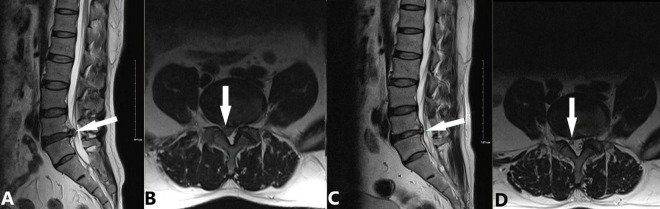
Application of targeted nucleus pulposus chemical ablation for LDH caused by extrusion at the right posterior margin of L4/5. A 45-year-old male patient presented with a 3-month history of lumbar distension and pain, which had progressively worsened in the past week. Considering the extruded nucleus pulposus and its moderate volume, we employed the CT-guided local targeted treatment method proposed by Wu et al. (8). The procedure involved the following steps: (1) A right posterolateral approach to puncture into the anterior epidural space; (2) Targeted injection at the extruded nucleus pulposus, including extradiscal injection of 600 U collagenase (WeiBang Biopharm, Liaoning, China) and 6.5 mL of an anti-inflammatory analgesic solution (0.5 mL betamethasone, 1 mL vitamin B_12_, and 5 mL 0.25% lidocaine); (3) No intradiscal injection of collagenase or oxygen-ozone mixture. (A,B) Preoperative images showing L4/5 disc protrusion (white arrow) compressing the dural sac and causing spinal stenosis. The right L5 nerve root is obscured by compression (A: sagittal view, B: axial view). (C,D) 90-day follow-up images showing complete resorption of the protrusion and decompression of the dural sac. The right L5 nerve root is clearly visible (white arrow; C: sagittal view, D: axial view).
